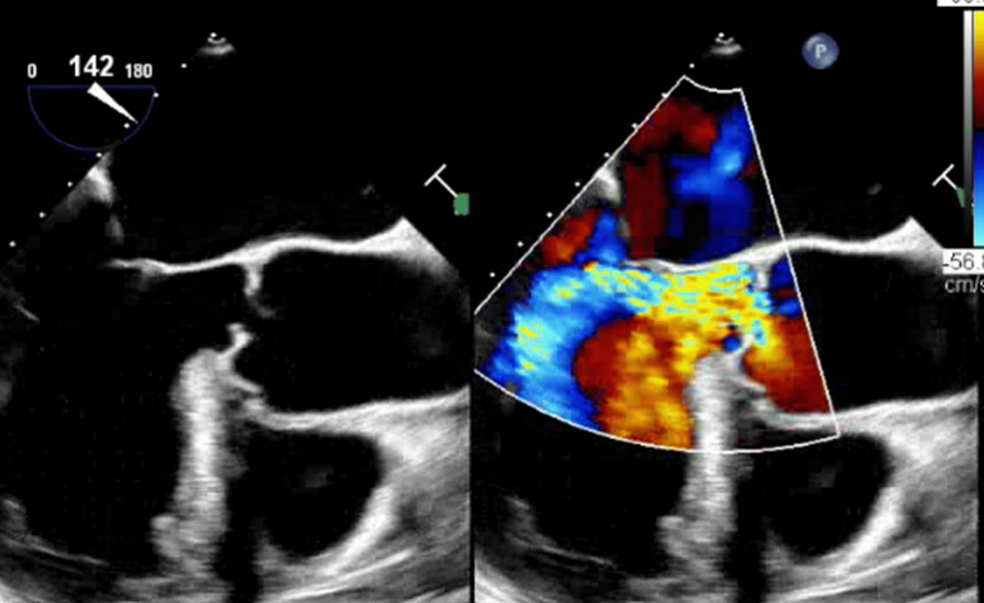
Aortic valve seen on a TEE, demonstrating severe AI and AVP AI, aortic insufficiency; AVP, aortic valve prolapse; TEE, transesophageal echocardiogram
